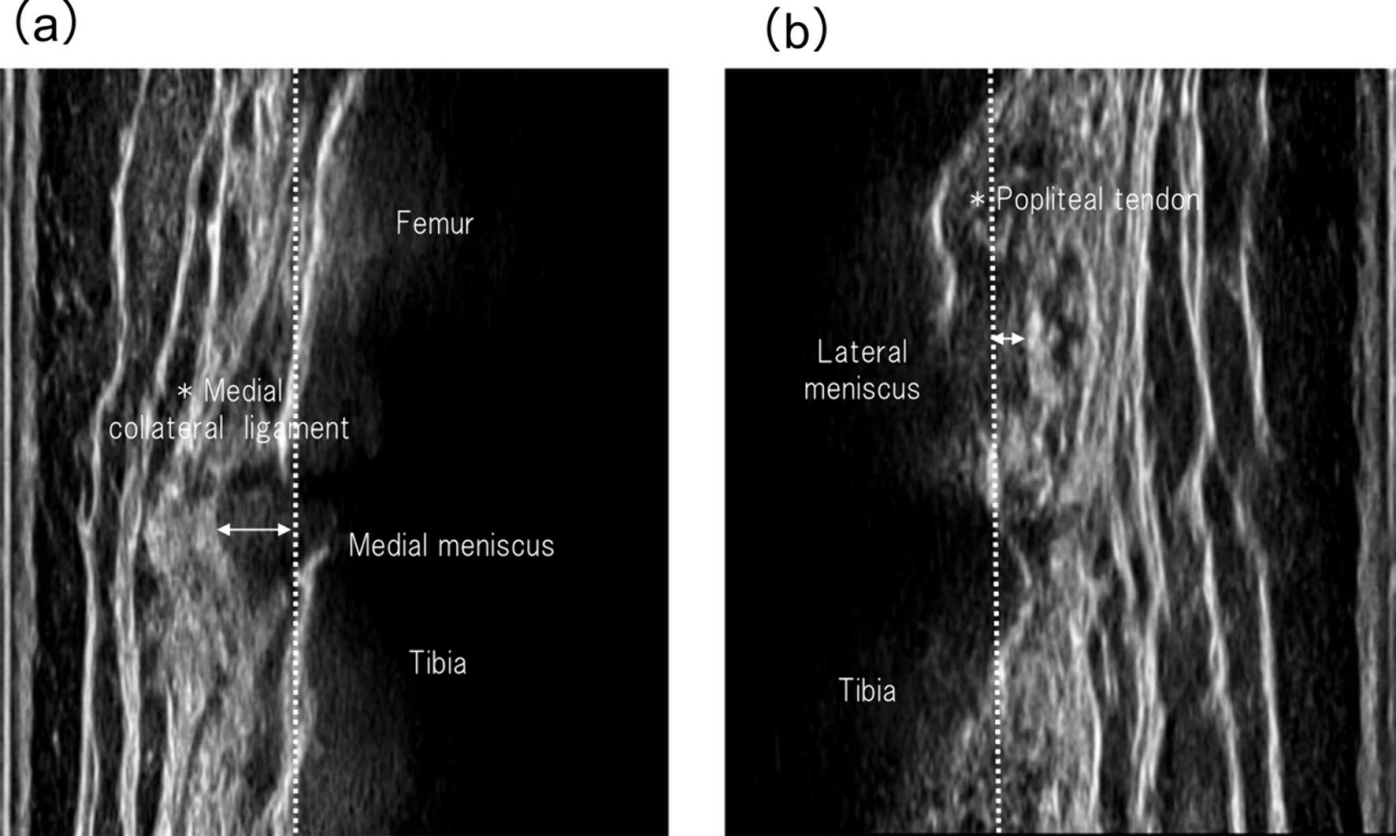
Representative ultrasound images. Coronal images depict the medial a and lateral b menisci, with * indicating the medial collateral ligament and popliteal tendon as landmarks. White dashed lines represent the reference line based on the tibial plate and arrows indicate the extent of meniscal extrusion
